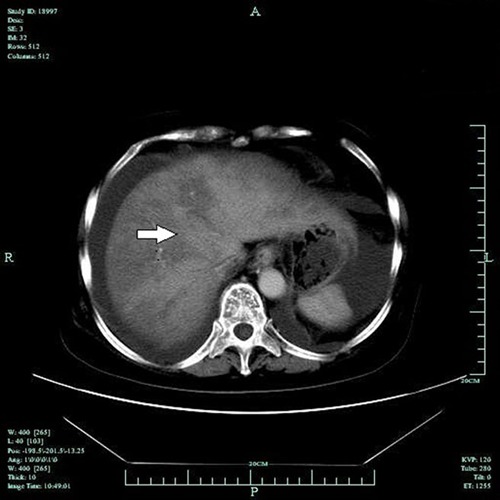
Unenhanced CT image of the liver of a patient with hepatic veno-occlusive
